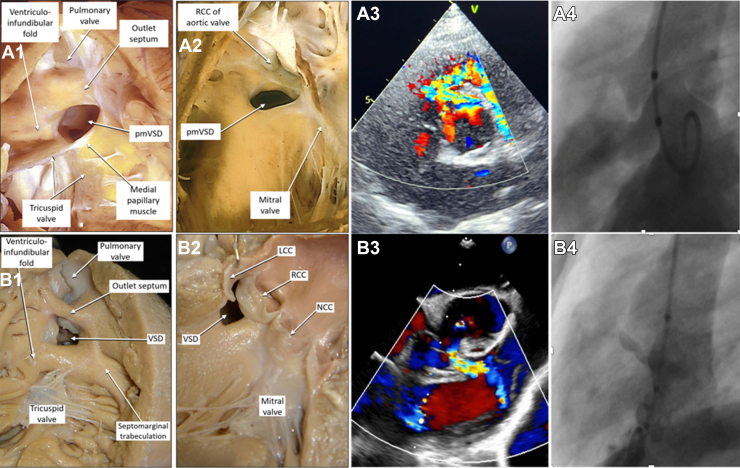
Comparison of Outlet pmVSD and Isolated Subaortic (Muscular Outlet) VSD (A1-A4) Outlet pmVSD. (A1, A2) pmVSD with outlet extension located below the outlet septum, maintaining separation from the pulmonary valve. (A3) Transthoracic echocardiogram. Parasternal short-axis view showing a large outlet pmVSD extending from TV to more than half of the RCC of the aortic valve; (A4) LV angiography showing large pmVSD extending to subaortic with mild prolapse of RCC. The shunt goes down to the RV and away from the pulmonary valve. (B1-B4) Isolated subaortic VSD. (B1, B2) Subaortic (muscular outlet) VSD with RCC prolapse. The VIF distances the defect from the tricuspid valve. (B3) On transesophageal echocardiogram, an isolated subaortic VSD is shown without perimembranous extension. The defect is located away from the septal leaflet, and the shunt jet is narrow and directed strictly anteriorly, pointing toward the right ventricular outflow tract (RVOT). (B4) LV angiography shows that the shunt is beneath the RCC and moves forward directly to the RVOT. LCC = left coronary cusp; other abbreviations as in Figures 1 and 2.
